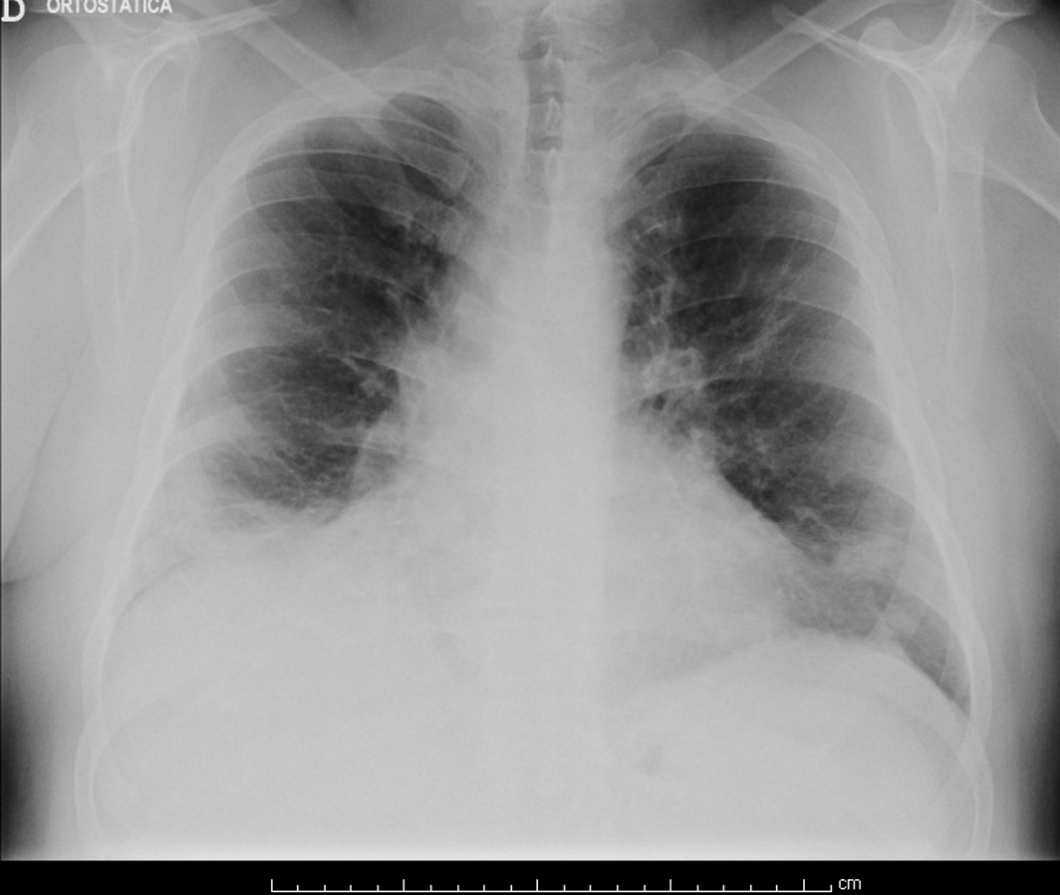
Chest radiography on discharge. An evident decrease in infiltrates can be observed over both lung fields.
